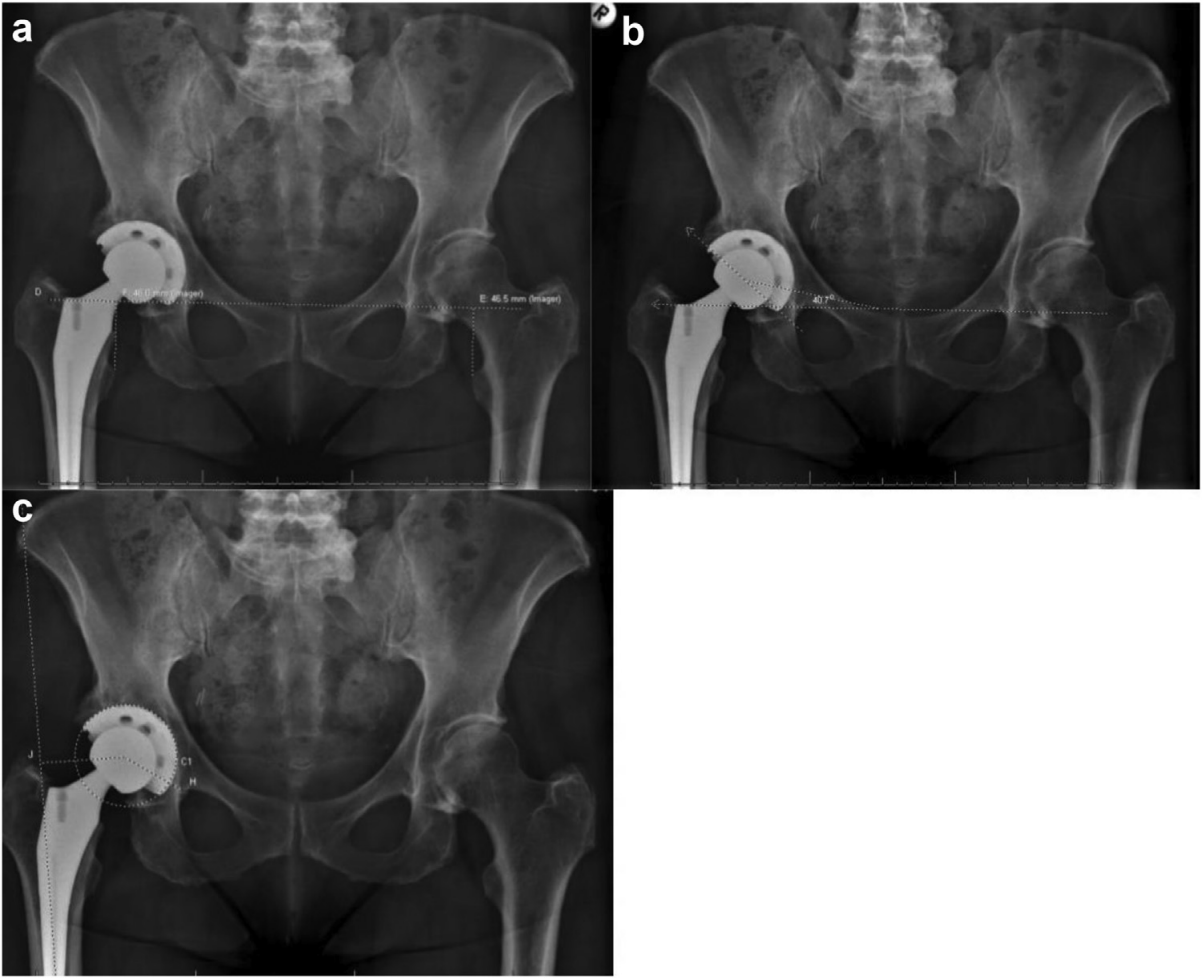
Post operative radiographs showing measurements of limb length (A), cup abduction (B), and hip offset difference (C).
